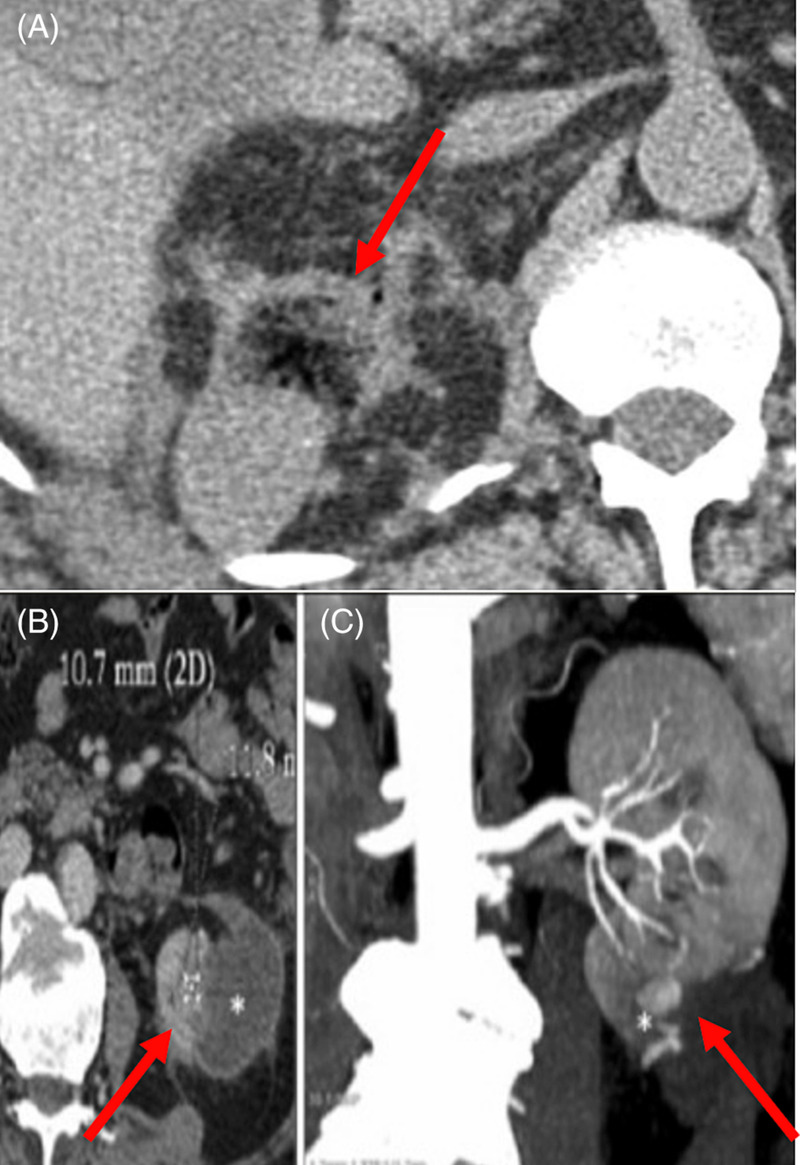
(A–C) Post‐partial nephrectomy surveillance computed tomography scans demonstrating local enhancement and air locules at site of resection (red arrows), when synthetic haemostatic bolsters have been used.
